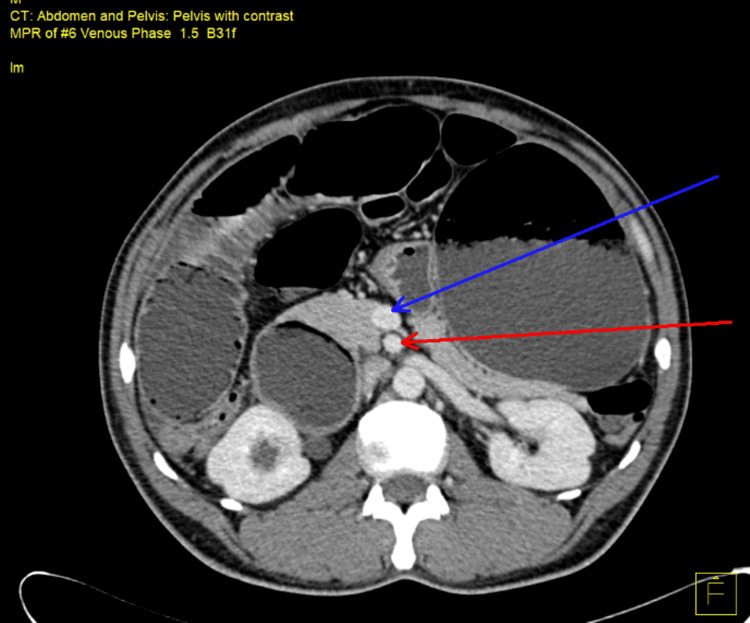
Axial view of venous phase of contrast enhanced CT scan of abdomen and pelvis showing superior mesenteric vein (blue arrow) and superior mesenteric artery (red arrow)
